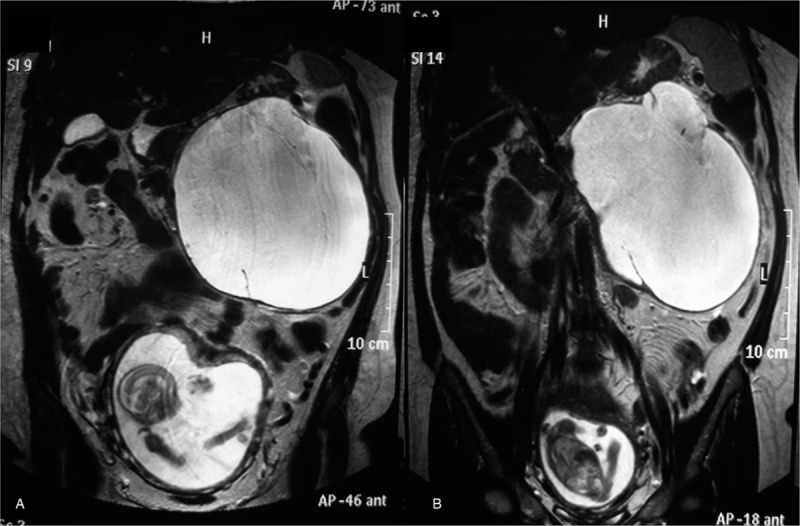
Magnetic resonance imaging showing a 20 cm × 18 cm × 18 cm cystic neoplasm arising from the pancreatic tail during pregnancy.
